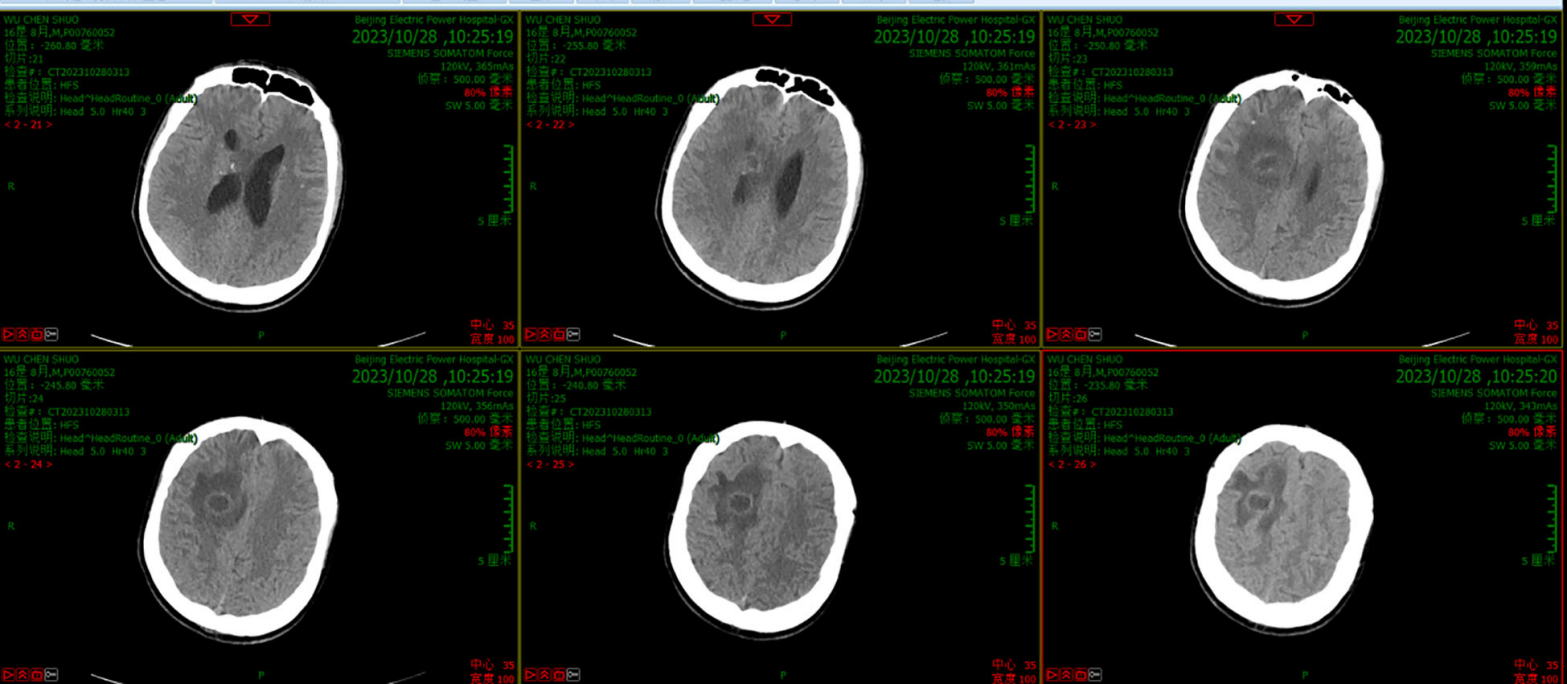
CT scanning before the new therapeutic regimen showed a round cystic-solid lesion in the operative area, with the interior of the lesion being hypointense and the surrounding solid cystic wall being hyperintense. The brain tissue around the lesion was evidently edematous.
